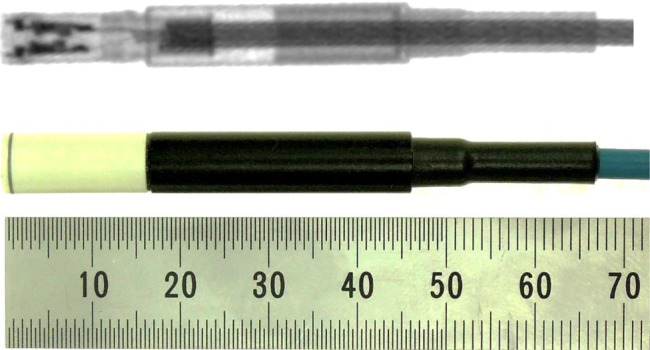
Photo and X-ray image of the PTW Type 60019 detector.
